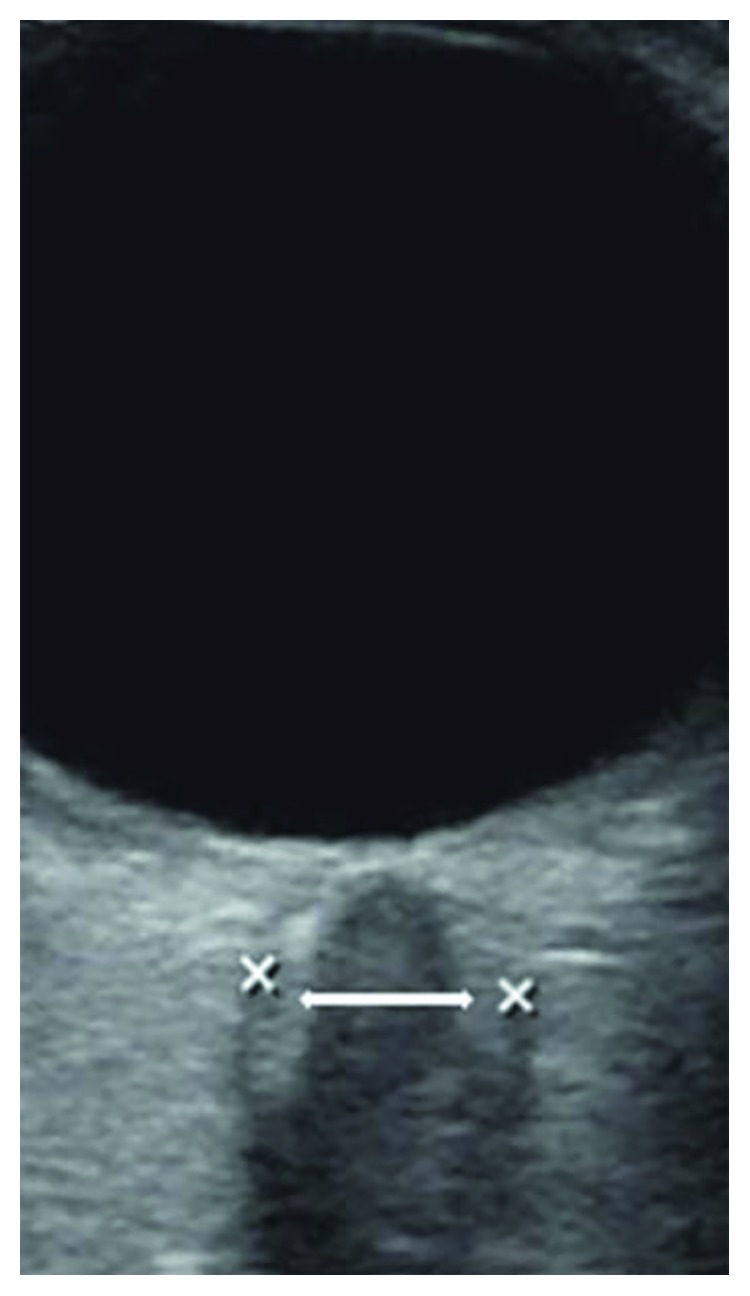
Employment of the “black stripe” method (white arrow) may underestimate the actual optic nerve sheath diameter (ONSD) measurement (*x*–*x*) based on the new sonographic quality criteria in patients with increased intracranial pressure.
